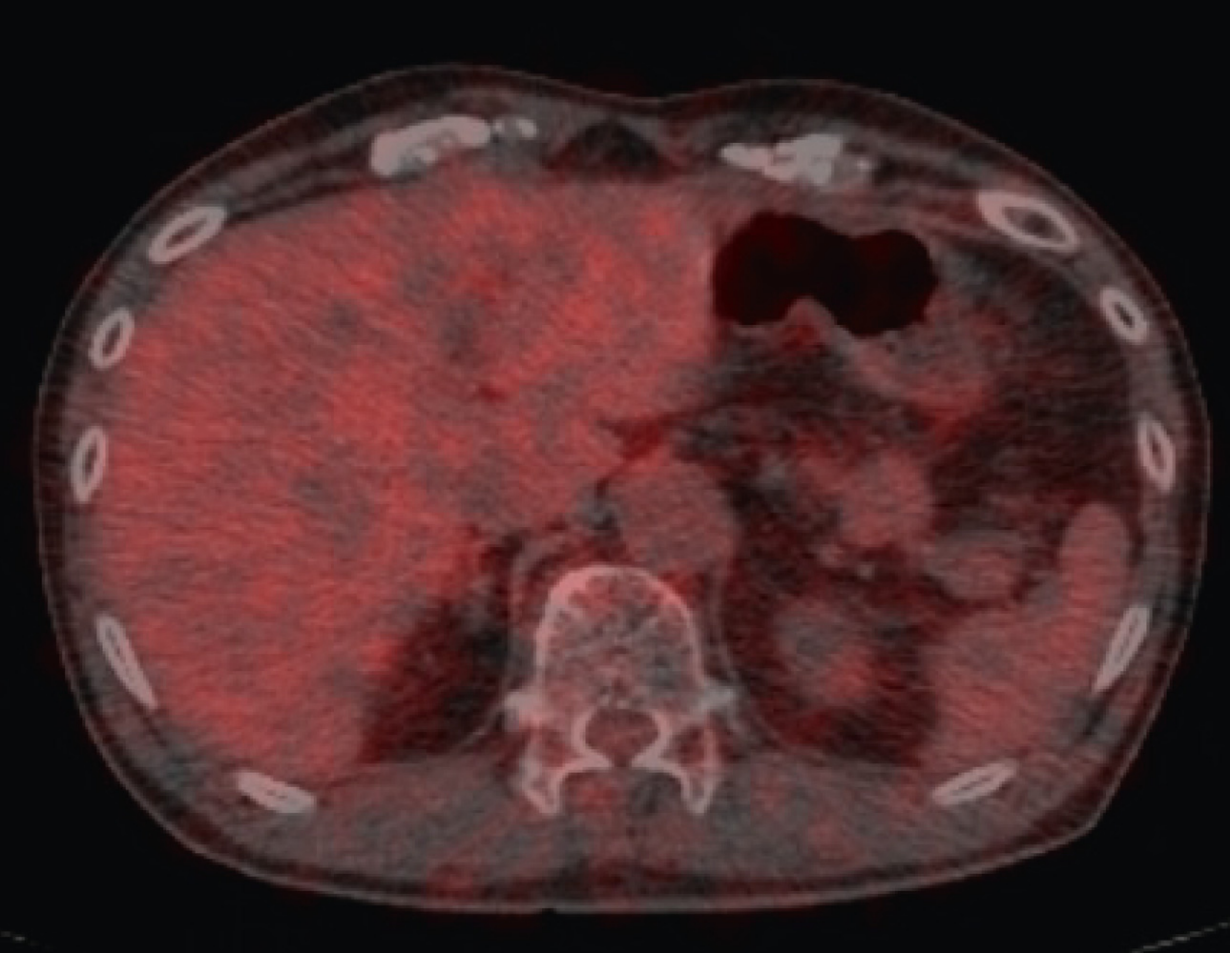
PET/CT showed no abnormal uptake.
